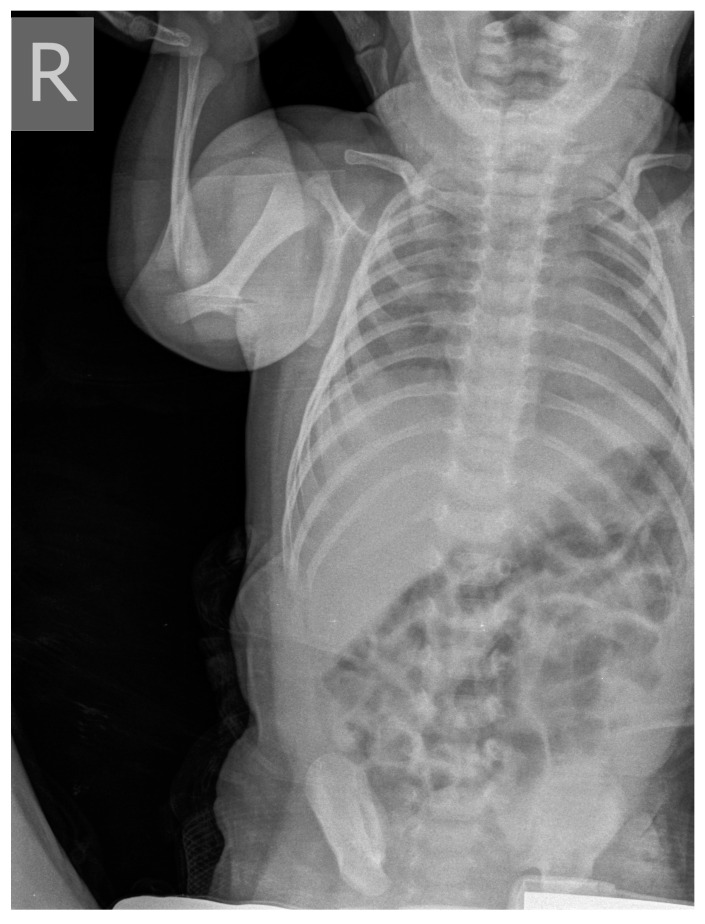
X-ray of thoracic in neonate 2 on the 33rd day of hospitalization: On the right, in the upper and medial lung fields, the transparency of the lung parenchyma is inhomogeneously reduced. On the left, the lower lung field is in superposition with heart shadow. The hemidiaphragms of clear contours.
